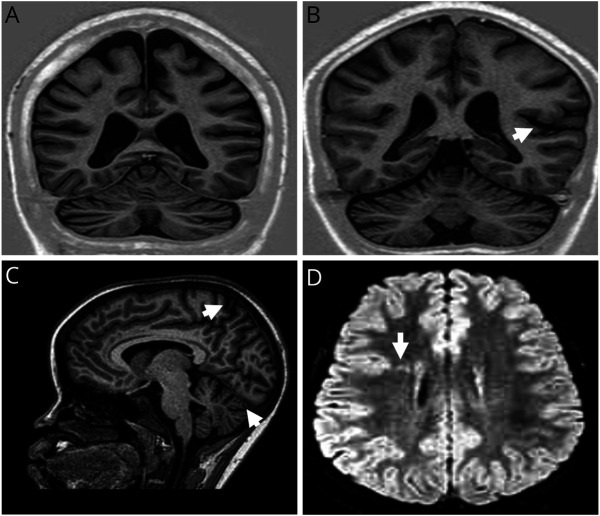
MRI of Participants With NS and OAE (A) 16-year-old girl with NS with global cerebral and cerebellar atrophy (T1- weighted inversion recovery). (B) 15-year-old boy with NS with left temporal lobe atrophy and bilateral cerebellar atrophy (white arrow showing the temporal lobe atrophy) (T1-weighted inversion recovery). (C) 14-year-old girl with OAE with cerebellar atrophy and global cerebral atrophy (small white arrow) (T1-weighted). (D) 17-year-old girl with OAE with type 2A focal cortical dysplasia in the inferior right frontal lobe (white arrow) (T2-FLAIR). Abbreviations: FLAIR = fluid-attenuated inversion recovery; NS = nodding syndrome; OAE = onchocerciasis-associated epilepsy.
